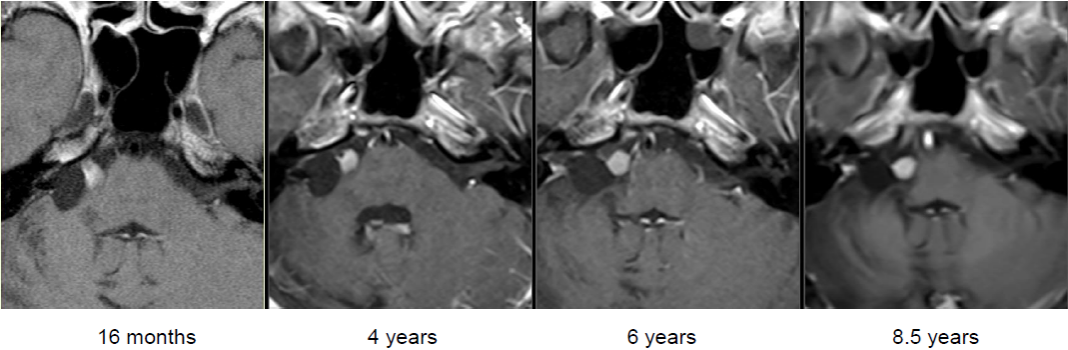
Up to now, the postoperative MRI follow-up (T1-w + CM, axial) did not show any growth of the residual tumor.
